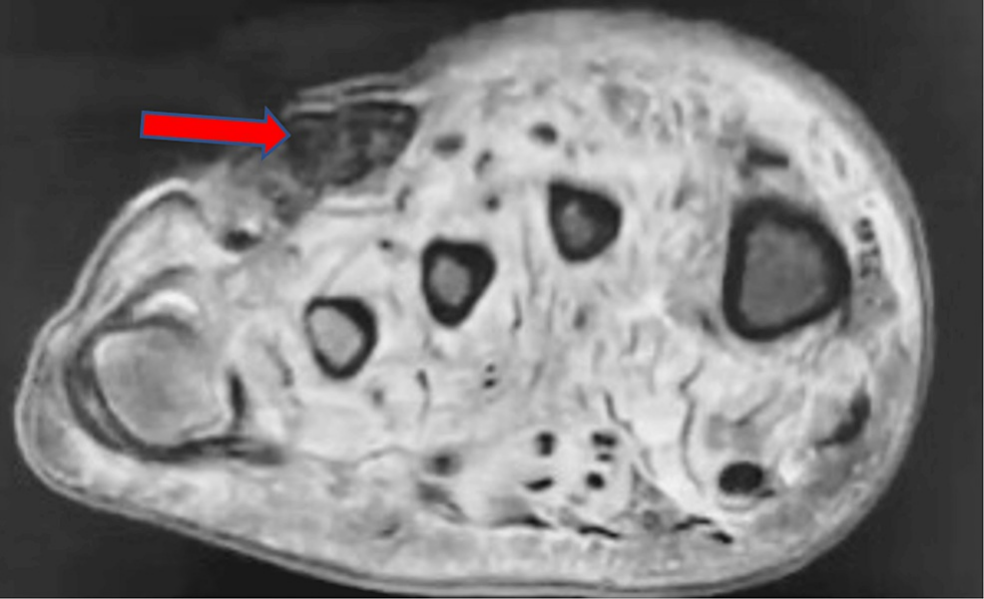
Axial MRI Without Contrast of the Right Foot Axial MRI Without Contrast of the Right Foot showing ulcers in the dorsum of the forefoot with some tracking of the subcutaneous air communicating with the skin lesions (red arrow).
